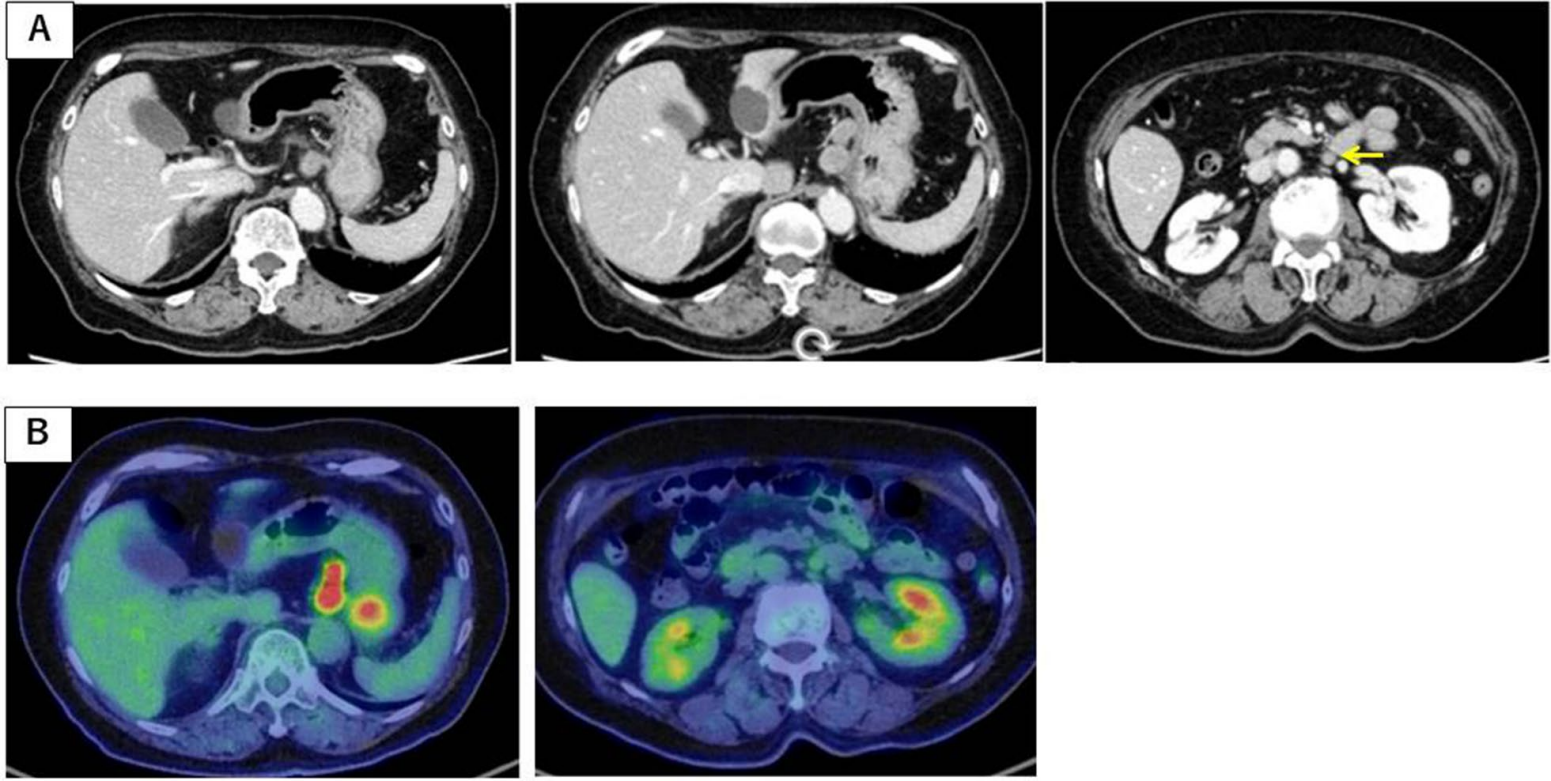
Computed tomography (CT) findings and 18F-fluorodeoxyglucose positron emission tomography (^18^F-FDG PET) CT imaging findings. A CT showed a mass approximately 3 cm in diameter in the gastric cardia, swollen lymph nodes along with the lesser curvature and para-aortic lymph node (arrow). B
